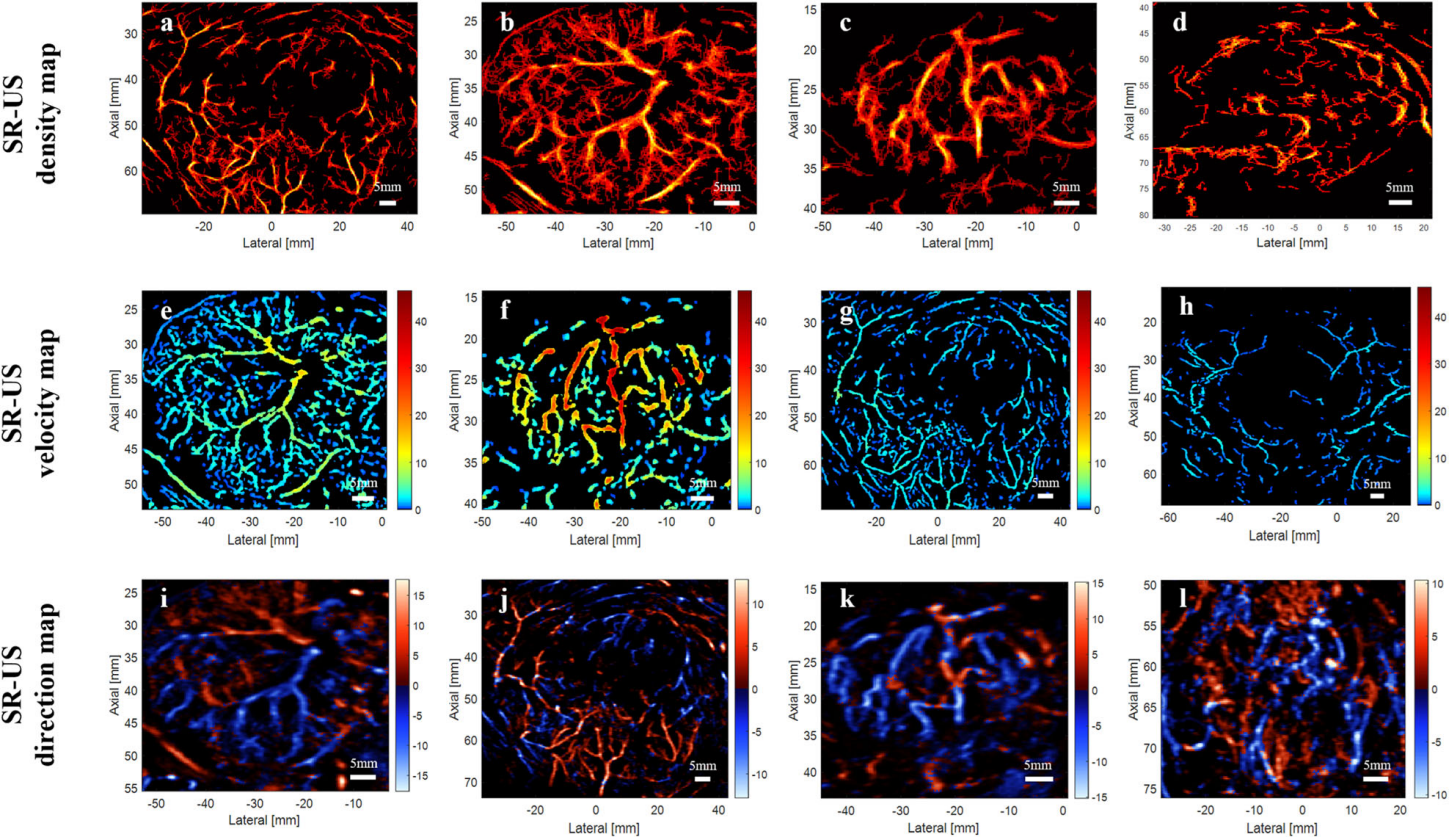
Examples of different super-resolution ultrasound (SR-US) parametric patterns. Density maps: a peripherally distributed pattern; b well-distributed pattern; c, d irregularly distributed pattern. The color bar corresponds to the normalized number of localized microbubbles. Velocity maps: e, f high-speed feeding pattern; g, h low-speed supplying pattern. The color bar corresponds to different speeds. Direction maps: i centrifugal pattern; j centripetal pattern; k eccentric pattern; l mixed pattern. The color bar represents the blood flow direction; red and blue indicate flow toward and away from the transducer, respectively. The white horizontal scale bar on all images represents 5 mm. a, g and j are images of a 51-year-old female patient with liver metastasis; b, e, i of a 35-year-old male patient with focal nodular hyperplasia; c, f, k of a 71-year-old female patient with hepatocellular carcinoma; d of a 65-year-old female patient with hepatocellular carcinoma; h of a 45-year-old male patient with liver metastasis; l of another 65-year-old female patient with hepatocellular carcinoma
